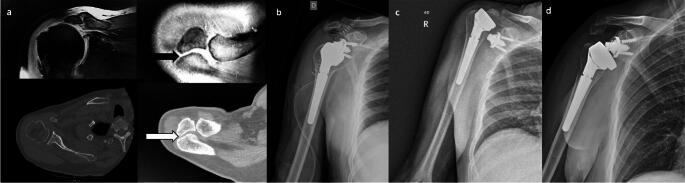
A 66-year-old patient with prosthetic instability (a) MRI: Rotator cuff arthropathy with os acromiale (mesoacromion), coronal and axial plane. CT: Axial plane on glenohumeral joint and os acromiale. (b) Immediate postoperative radiographic control. (c) Prosthetic dislocation at 6 weeks. (d) Radiographic control after revision surgery
